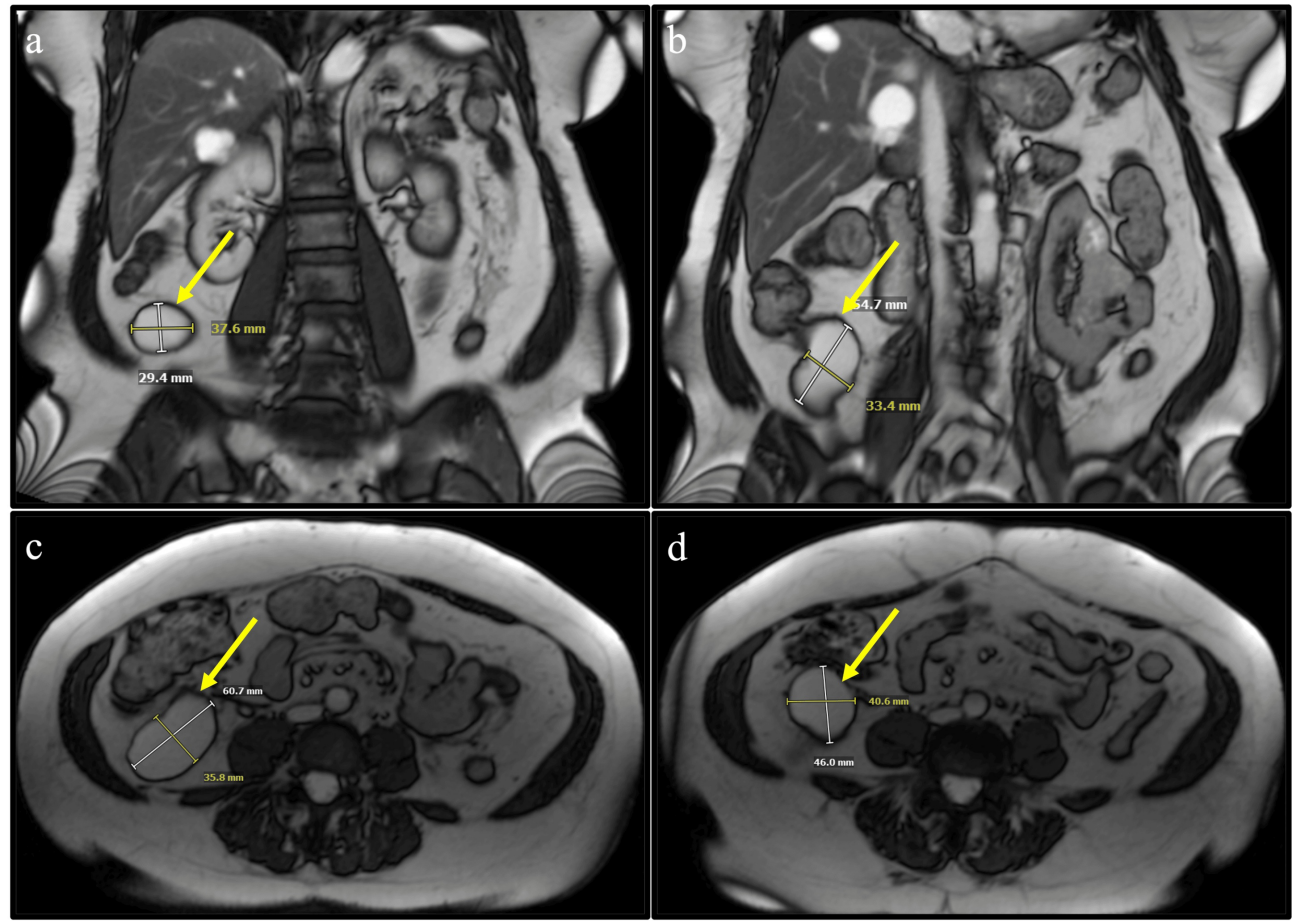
T2-weighted MRI highlighting the proposed appendiceal mucocele (a) coronal view of a posterior point of the cystic lesion; (b) coronal view of an anterior point of the cystic lesion. Note the continuity and attachment of the lesion to the appendix and/or cecum; (c) transverse view of an inferior portion of the cystic lesion; and (d) transverse view of a superior portion of the cystic lesion. Note hyperintense distention of the appendix and bright mucin appearance. Findings suggest appendiceal mucocele rather than peritoneal duplication cyst.
